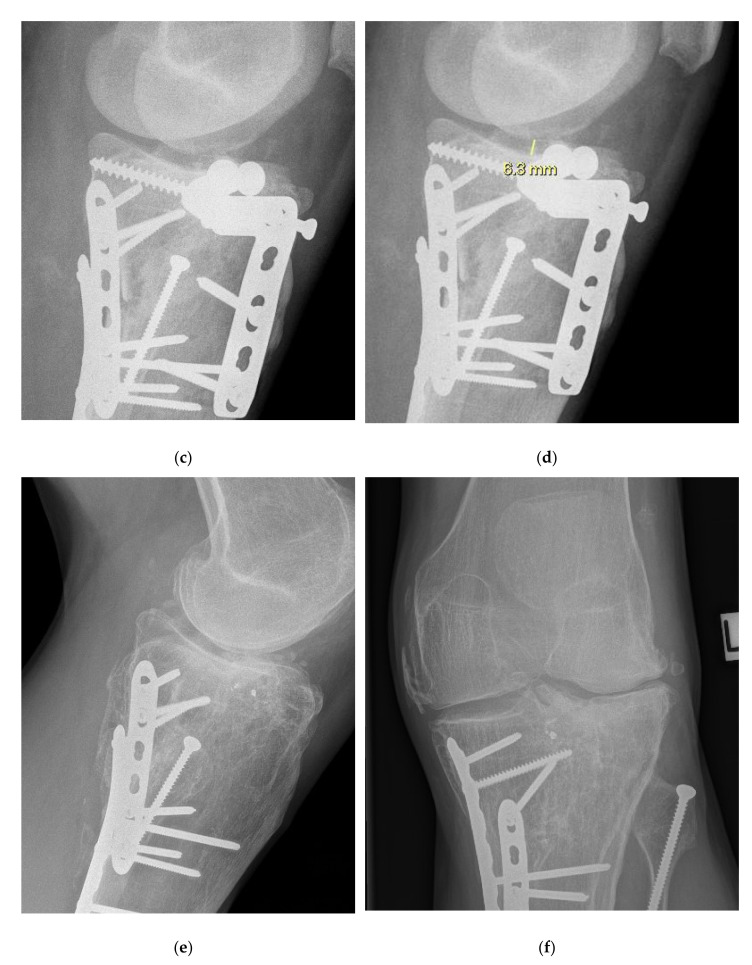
Pre- (a) and postoperative Day 2 (b) AP radiographs of a fracture that was not reduced (Schatzker VI) in a patient with Marfan syndrome. Note that the articular step is better visible on the lateral views (c,d). (e,f) Lateral and AP radiographs of the same fracture 5 years after injury following removal of the anterolateral plate and screws (removal of metalwork 21 months post primary surgery). Note the residual articular step and secondary degenerative changes. Despite fracture malreduction, the 5-year Lysholm score, KOOS pain score, and KOOS quality of life score were 80, 94.4, and 68.6, respectively.
